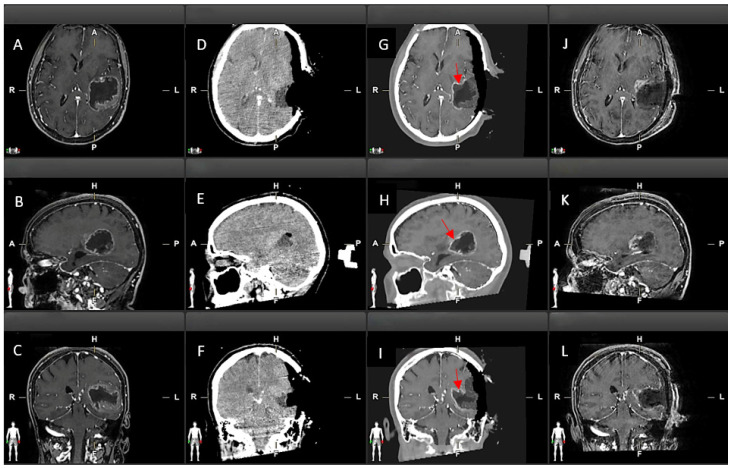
Method adopted for assessing the accuracy of Virtual iMRI. (A–C) Pre-operative MRI in axial, sagittal, and coronal view. (D–F) Post-contrast i-CT scan in axial, sagittal, and coronal view. (G–I) Virtual iMRI image with axial, sagittal, and coronal view. (J–L) Post-operative MRI in axial, sagittal, and coronal view. Red arrows indicate residual tumour on Virtual iMRI which is not included in the resection cavity (grey area).
